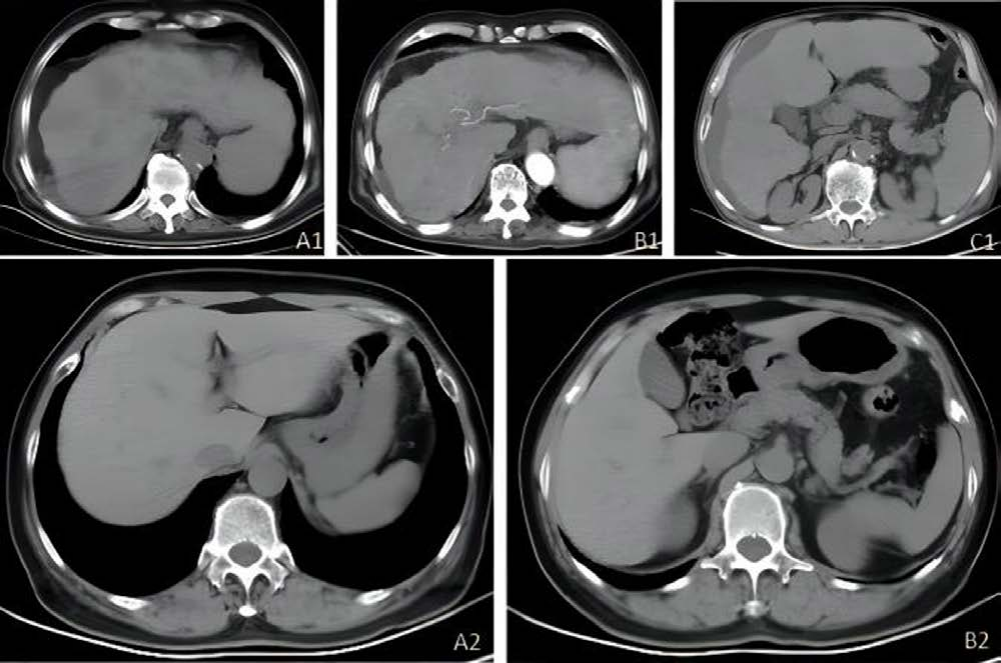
(A1, B1, and C1) Abdominal CT plain scan and enhanced scan of Case 2, indicating multiple liver nodules. (A2 and B2) Abdominal CT plain scan and enhanced scan of Case 3, indicating no significant abnormalities. CT = computerized tomography.
